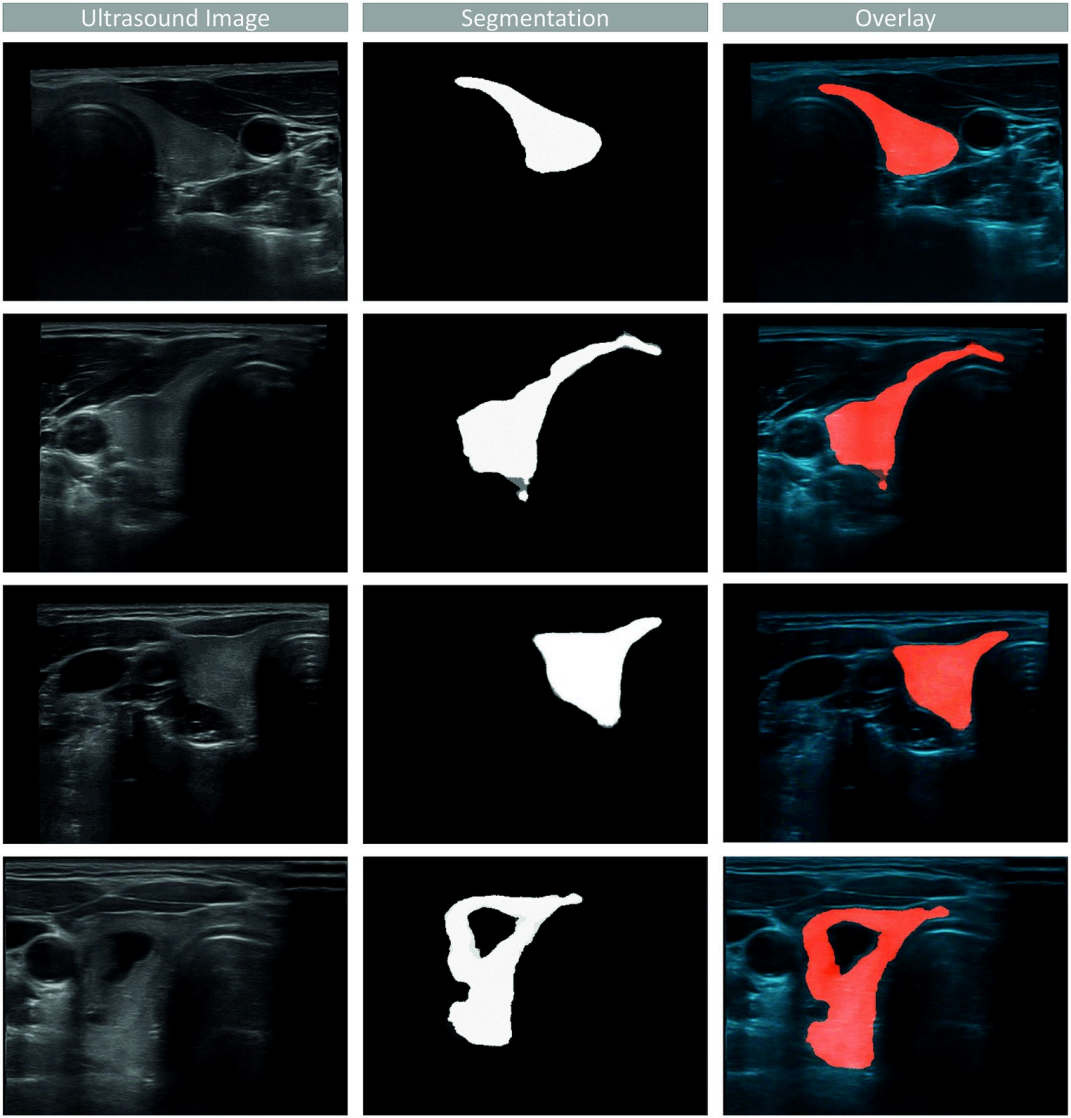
Ultrasound image (left), segmentation (middle) and overlay (right) of a good segmentation (first row), a segmentation which segmented too many voxels of the isthmus (second row), a segmentation with too few voxels of the isthmus segmented (third row) and a segmentation with a segmented nodule (fourth row).
